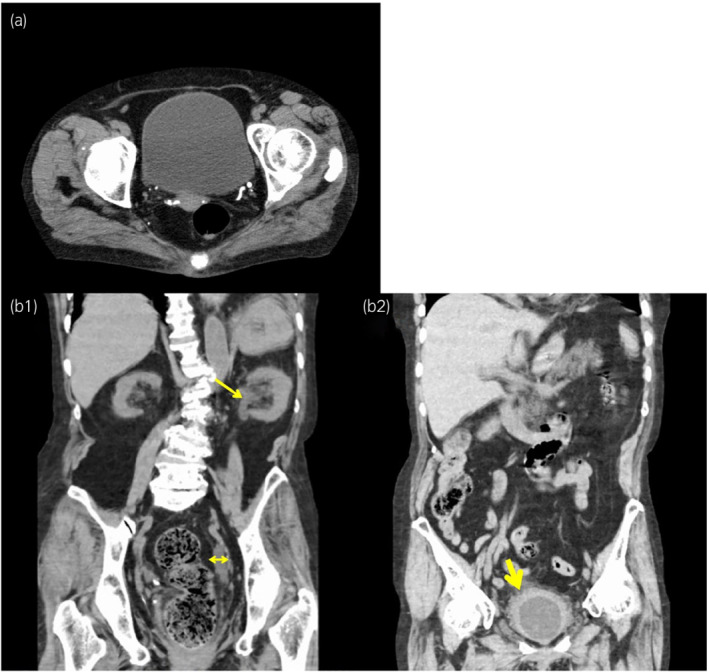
Serial CT images. (a) A CT image obtained at 9 months before BTX‐A injection showed no bladder wall thickness. (b) CT image obtained at 3 months after BTX‐A injection showed left hydronephrosis (arrow), ureteral dilatation (double‐headed arrow) (b1), and bladder wall thickness (big arrow) (b2).
